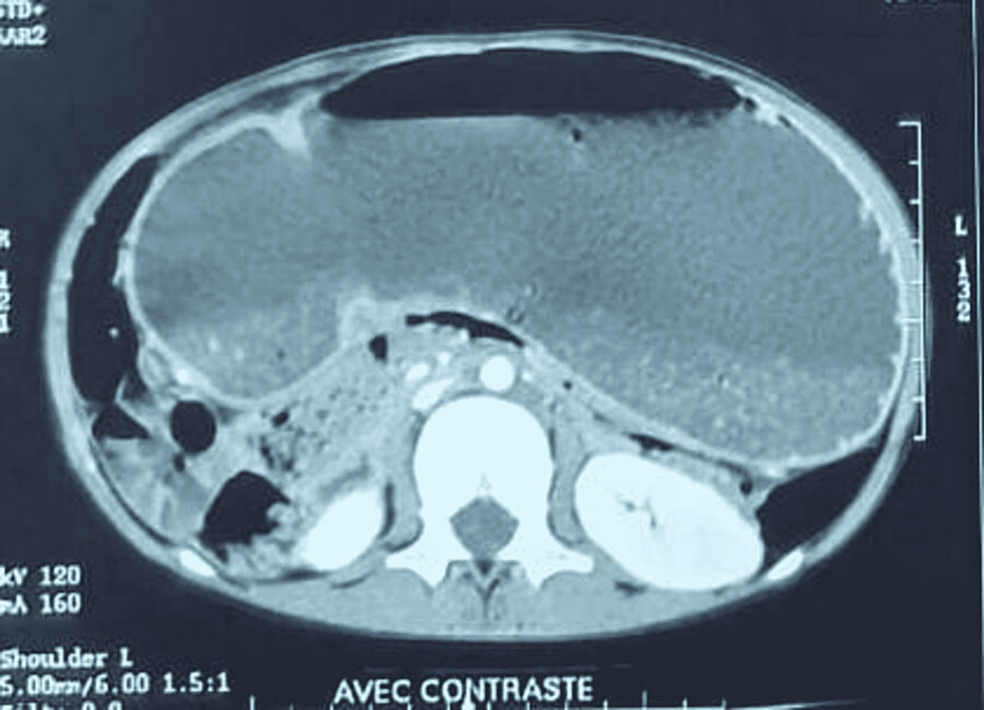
Abdominal computed tomography showing dilated stomach
